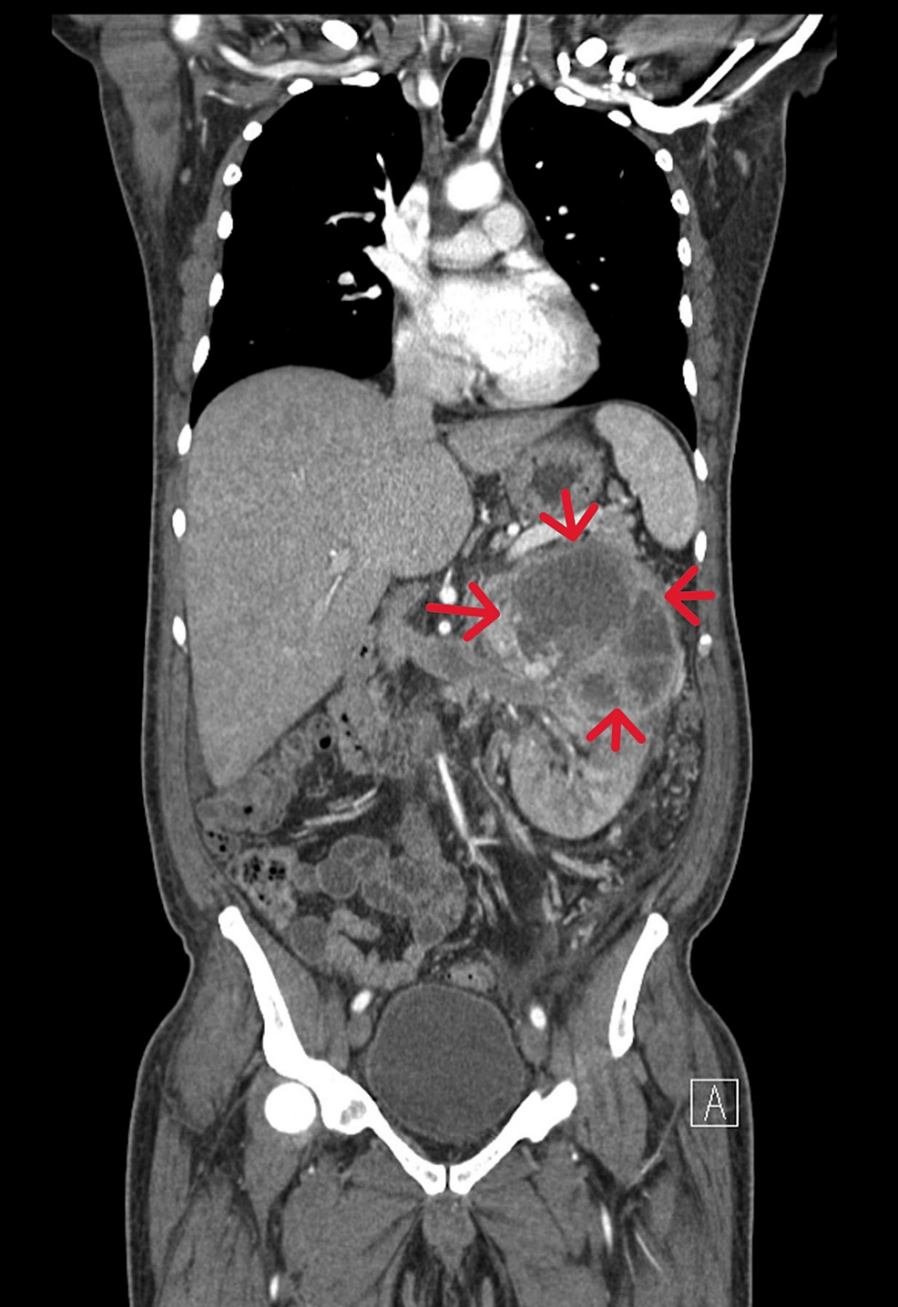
Mass in the right kidney viewed in the coronal plane on computed tomography (red arrows: renal mass in right kidney)
